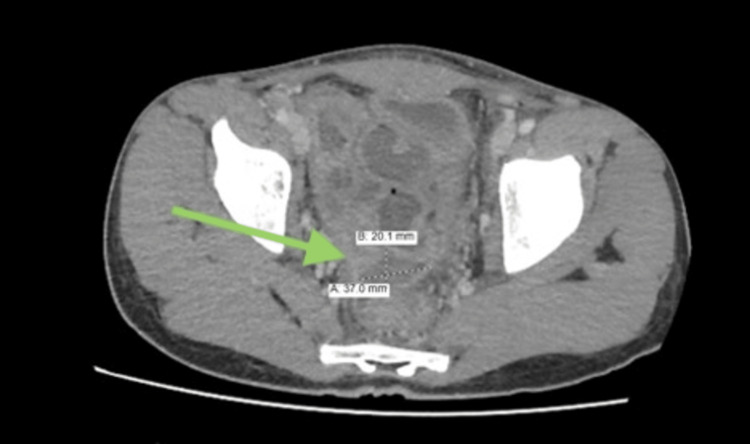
An axial CT image was obtained on readmission showing interval development of a multiloculated presacral fluid collection measuring approximately 15 mL, indicating intra-abdominal abscess formation.
